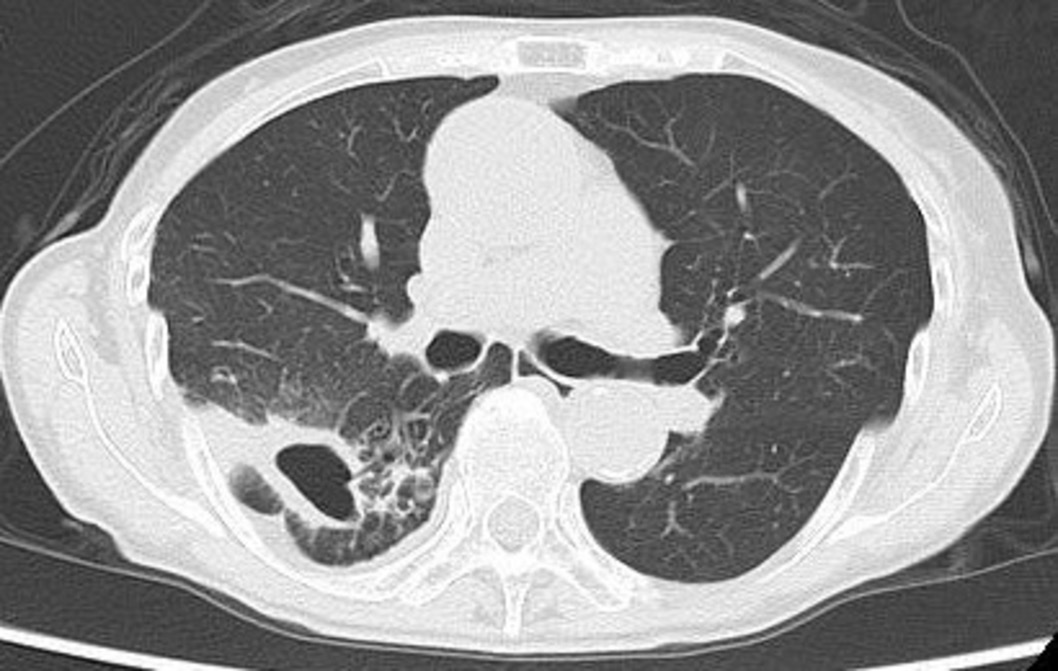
Chest computed tomography demonstrates a cavitary lesion formation in the right lower lobe.
